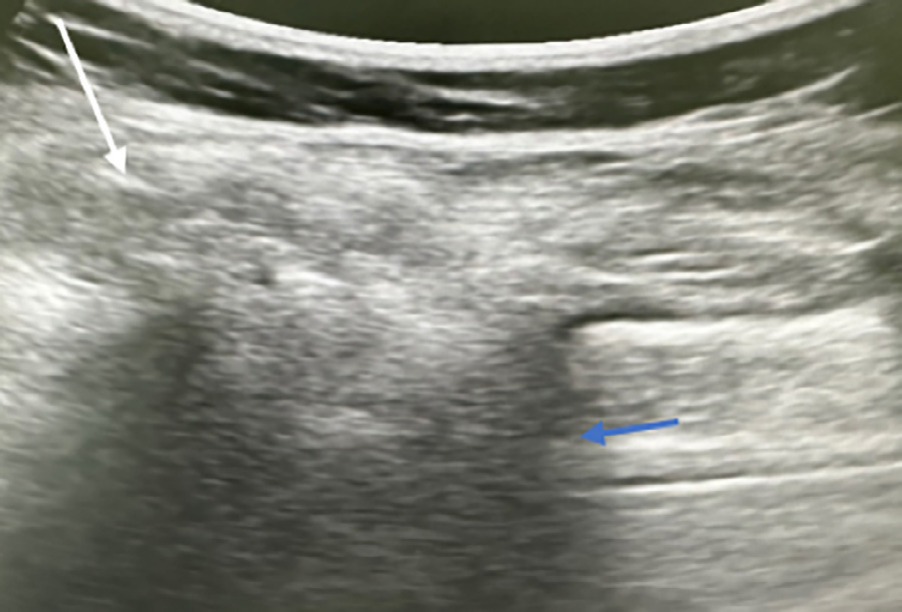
Ultrasound imaging shows a hernial sac containing omental and digestive structures (white arrow) with a wide neck (blue arrow).
